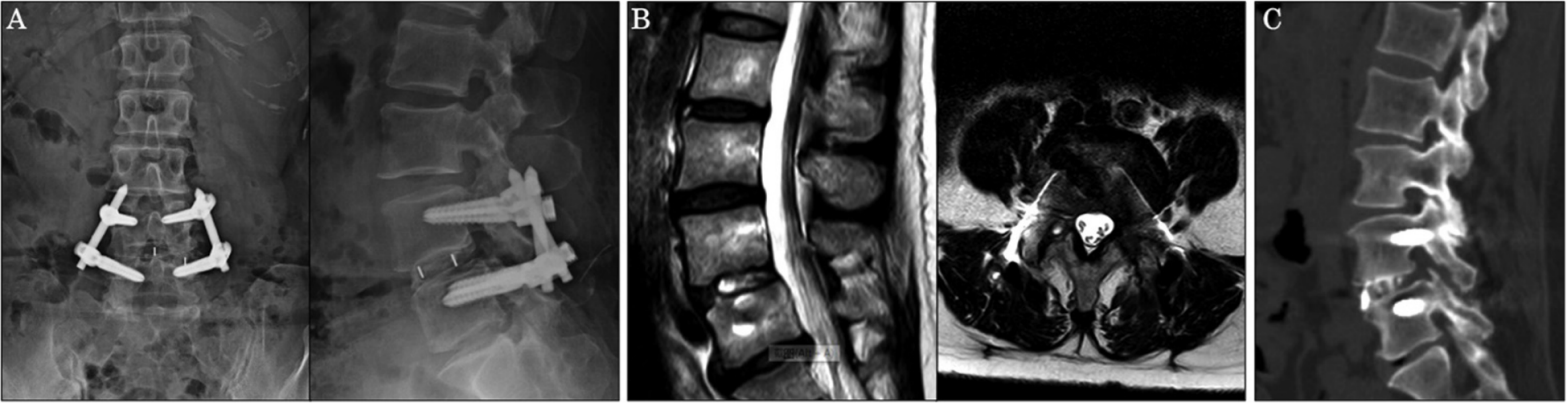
Images at 1-year post-surgery. a Anteroposterior and lateral X-ray films. Spondylolisthesis was corrected, and the hardware was in place. b Sagittal and axial magnetic resonance images. The spinal canal was decompressed without direct surgical decompression. c Computed tomography image showing the bone fused around the cage
